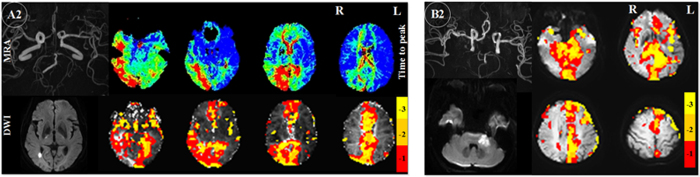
Perfusion injury in subacute stroke patients with severe stenosis/occlusion of the anterior cerebral artery (ACA), basilar artery (BA) and posterior cerebral artery (PCA). Hypoperfusion areas from time shift analysis (TSA) and time to peak (TTP) (A2), TSA (B2) maps were found in the corresponding blood supply areas of these arteries, although infarctions were only found in the right corona radiata, basal ganglia and occipital lobe (A2) and left brachium pontis (B2). MRA = magnetic resonance angiography, DWI = diffusion weighted imaging. −1, −2, −3 in color bar indicate −1TR, −2TR, −3 TR time shift.
